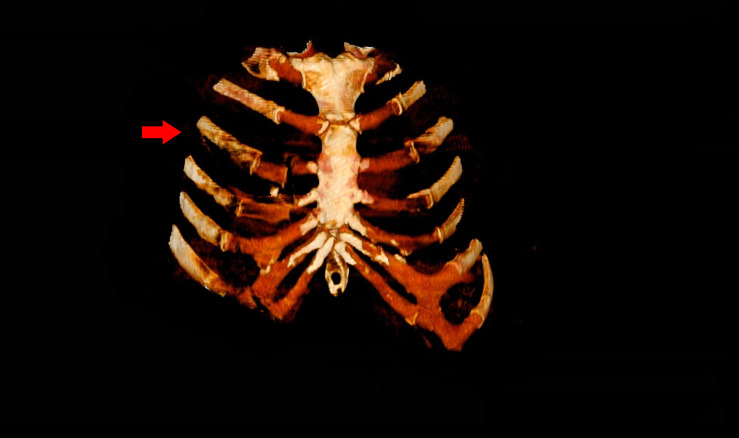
Three-dimensional reconstruction of the chest CT scan showing the dislocated costochondral fractures, starting at the right third rib (arrow).
